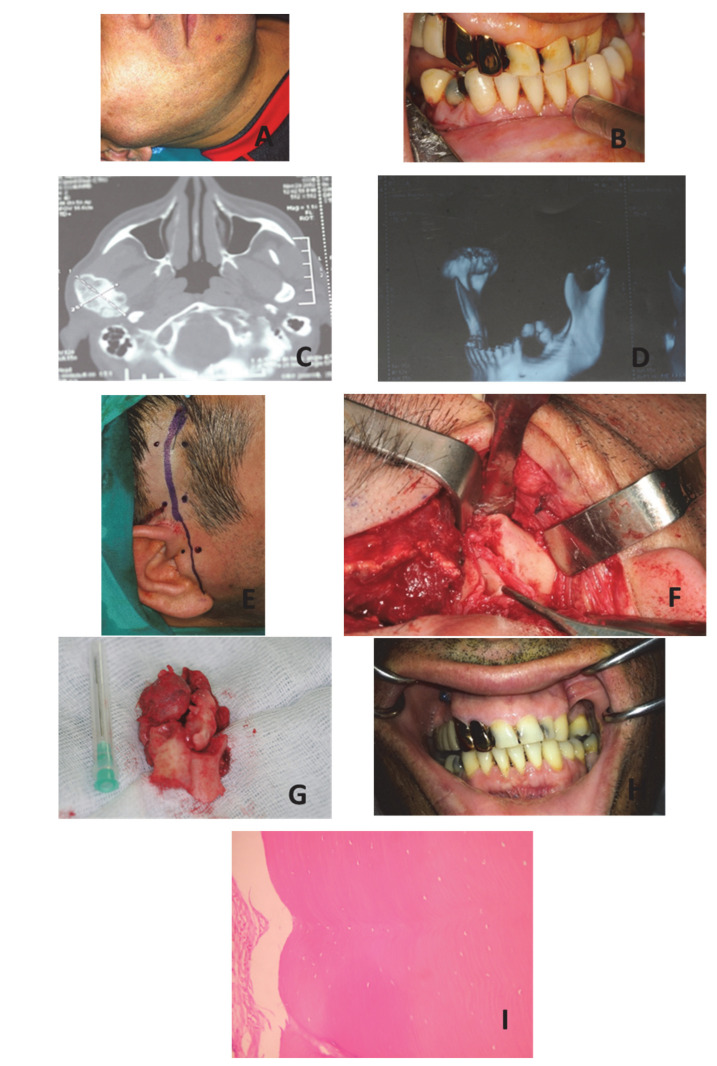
(A) Right pre-auricular swelling; (B) Posterior open bite on the right and transposition of the mandibular midline on the left; (C) Transverse view of CT showing the osteoma of the right mandibular condyle; (D) 3D reconstruction of the mandible showing the enlargement of the condyle; (E) Pre-auricular incision with temporal extension; (F) Exposure of the right mandibular condyle; (G) Osteoma after its extirpation; (H) The occlusion after removal of the osteoma; (I) Compact bone with very sparse arrangement of osteocyte and well-defined borders H & E X200.
